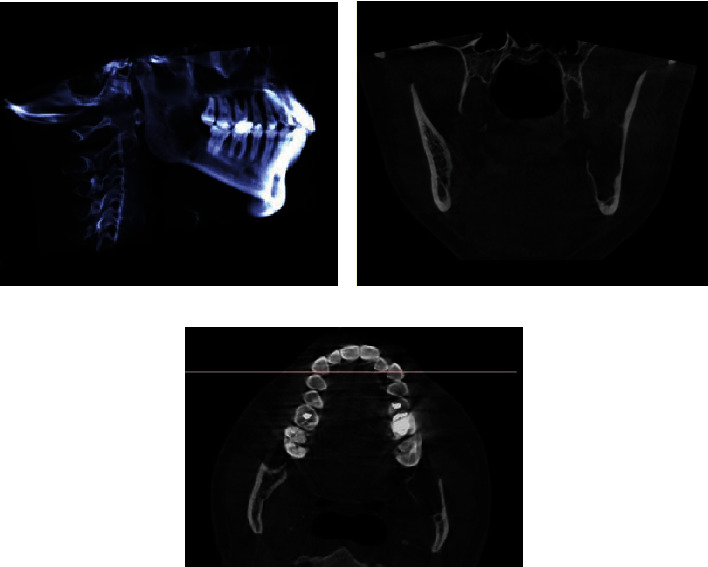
With computed tomography, the extension and involvement of the lingual cortical bone can be observed. (a) Sagittal view. (b) Coronal view. (c) Axial view.
